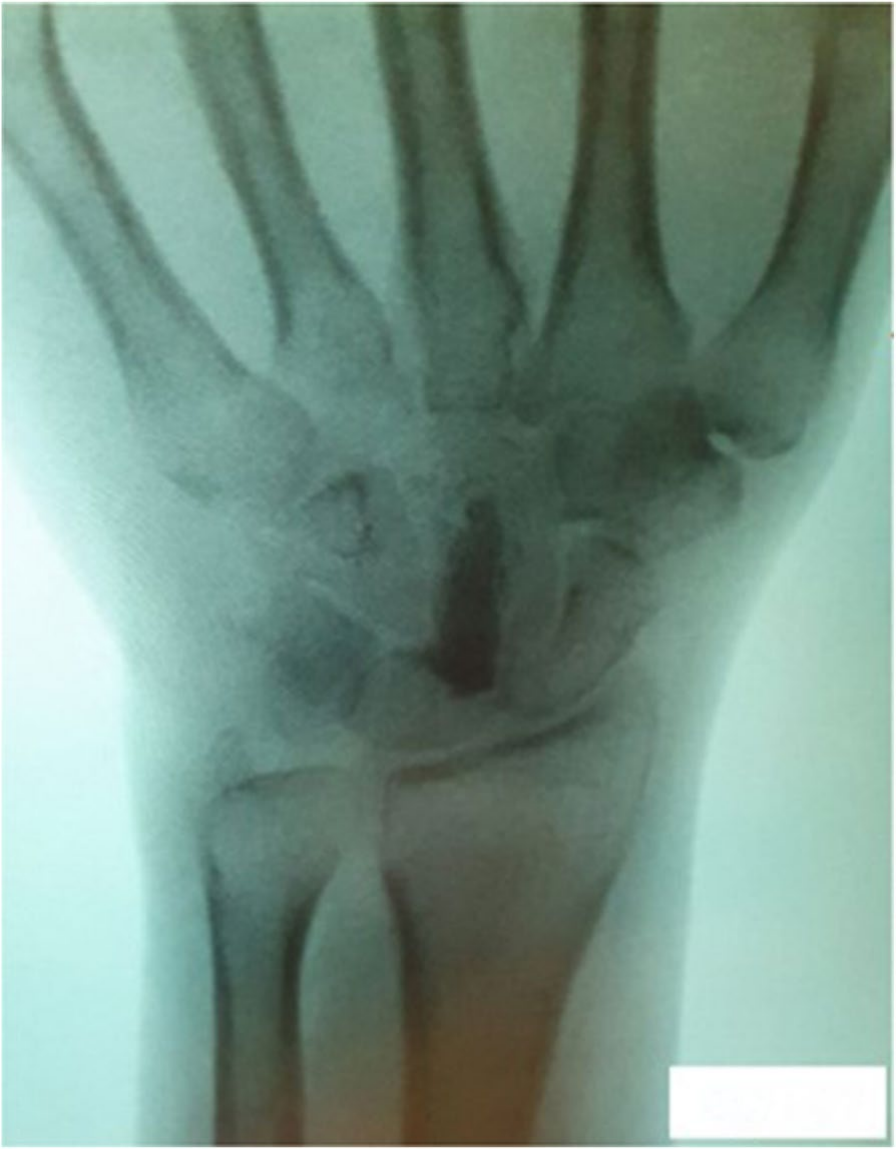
Preoperative plain radiograph showing an oval radio-opacity facing the carpal bones
